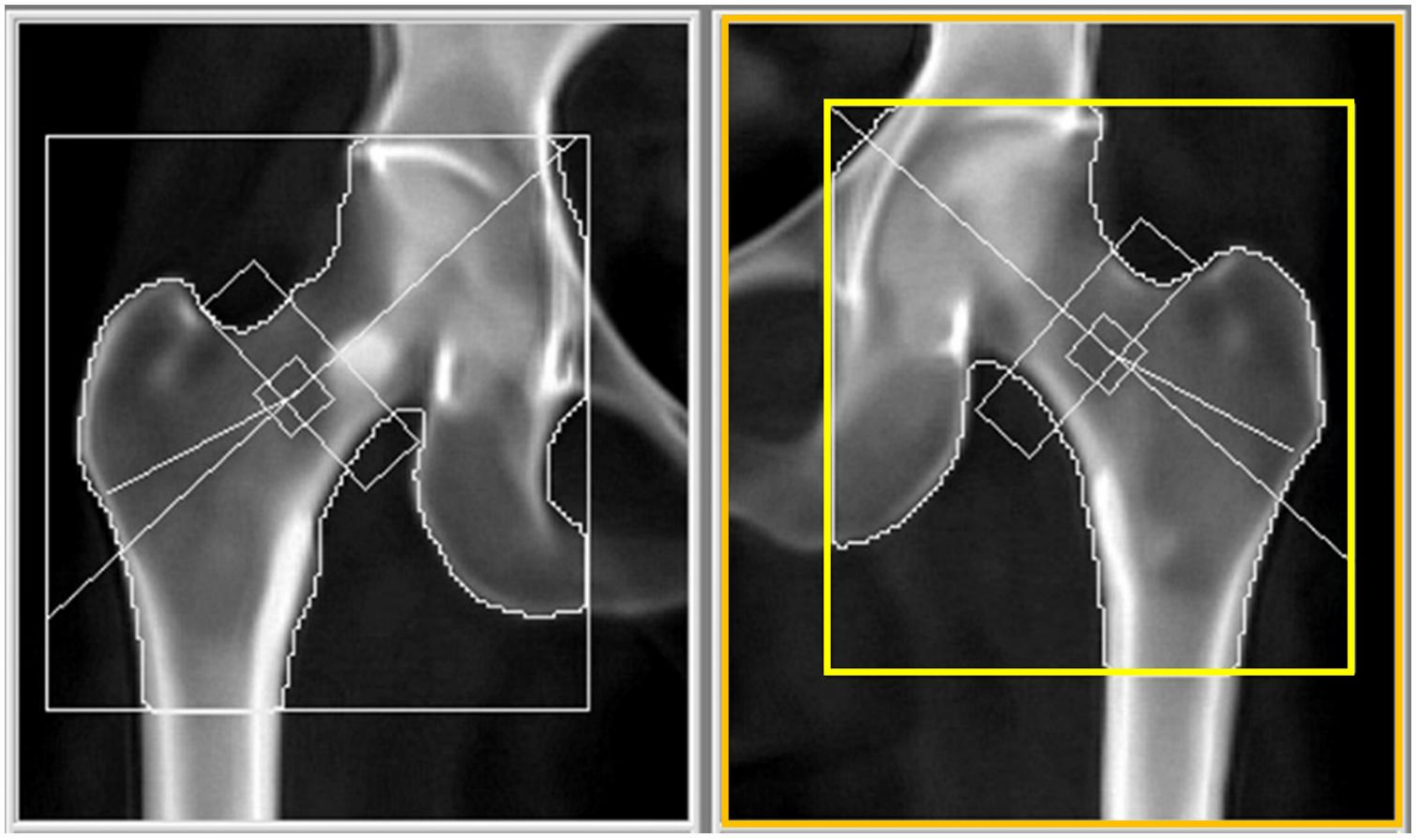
DXA scan conducted 1 month later as a callback, the T-score of left femoral neck recorded as -1.7, while d_0_ was 51.3. DXA = dual-energy X-ray absorptiometry.
